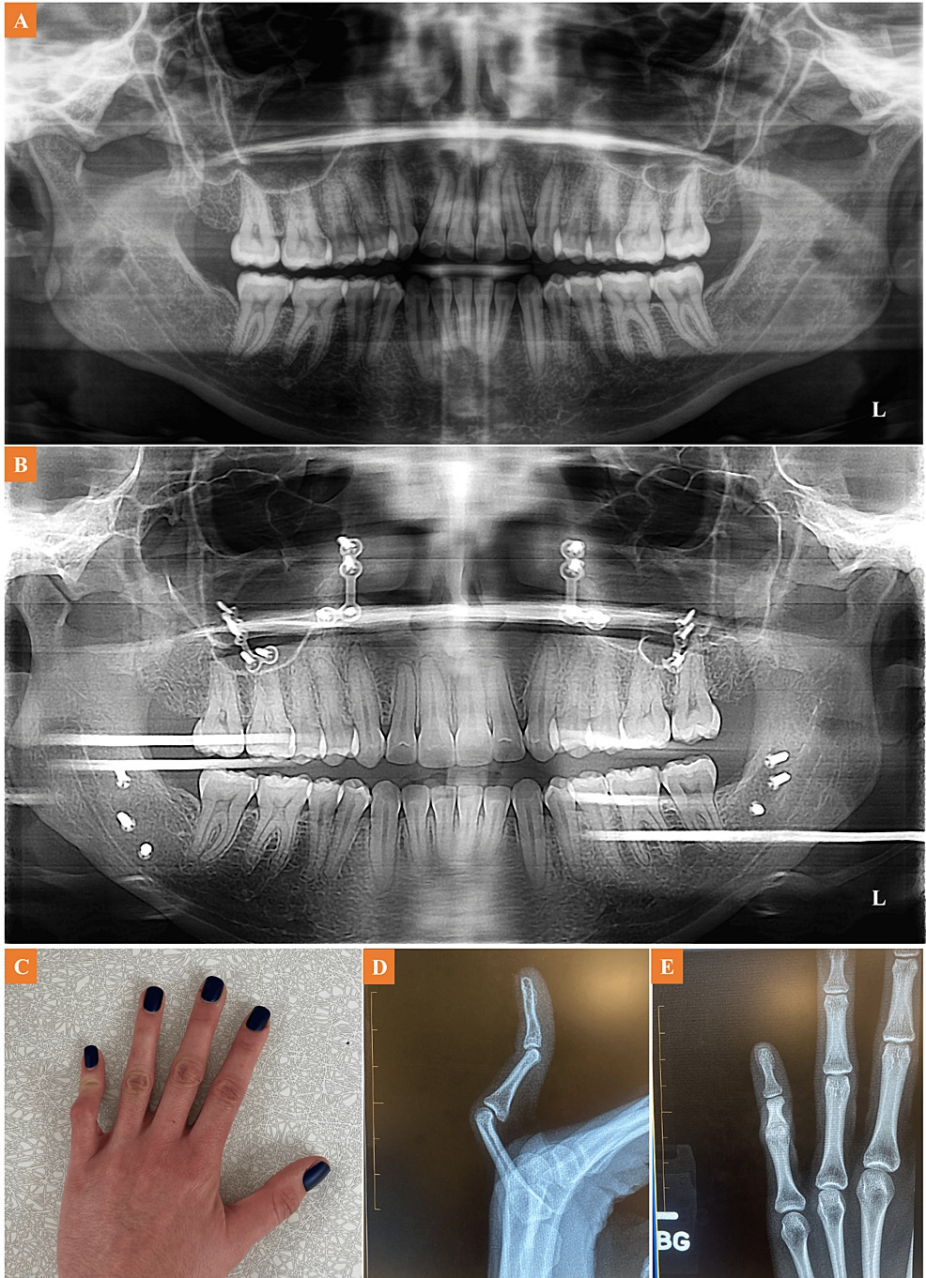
(A) Preoperative panoramic radiograph of the patient at 21-year-old; (B) post-operative panoramic radiograph of the patient at 22-year-old following LeFort 1 osteotomy and bilateral sagittal split osteotomy; (C) clinical picture of the left hand; (D) oblique radiographic view of the left hand; and (E) left hand posterior-anterior oblique radiographic view.
